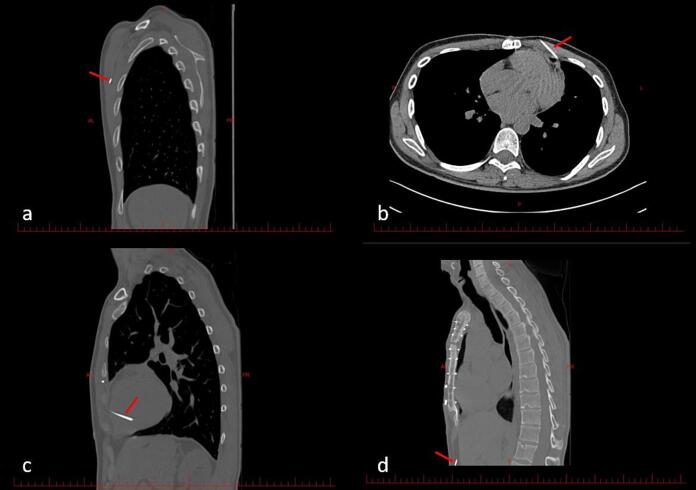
Axial and reformatted sagittal images of chest CT scan show a needle in the LV chamber (c) and three needles in the anterior thorax (a, b) and abdominal wall (d). red arrows show needles.
